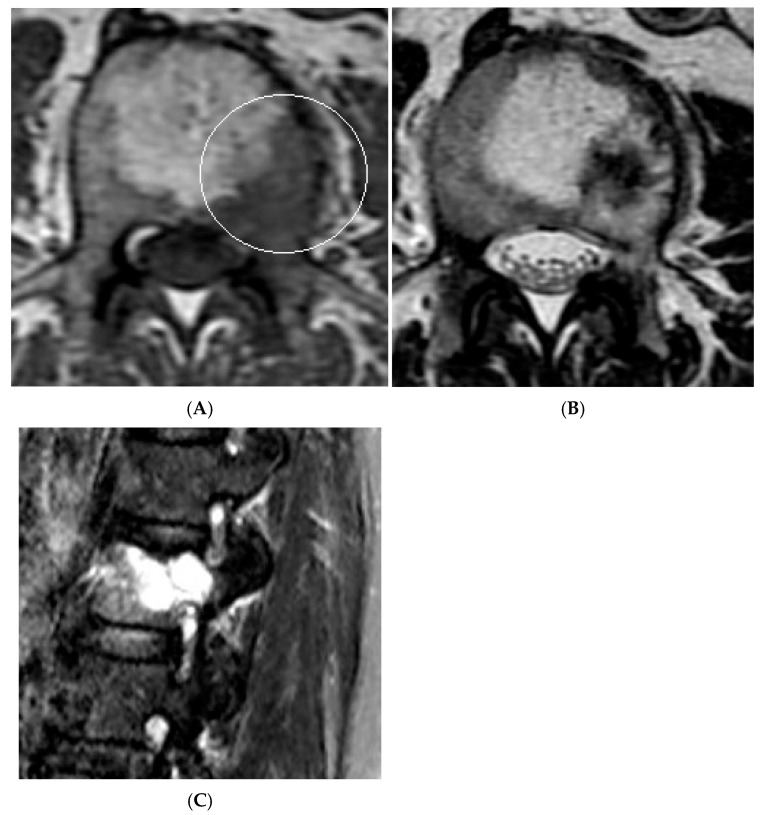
Collision Lesions—Renal cell carcinoma metastases within a haemangioma of the vertebral body, Case 2. (A) Axial T1W MRI with a typical vertebral body haemangioma with an abnormal low T1 signal in its posterolateral aspect with periosteal change (circled). (B) Axial T2W MRI demonstrates the aggressive lesion within and adjacent to the typical haemangioma in image (A). (C) Sagittal STIR MRI—Fat-suppressed haemangioma with a hyperintense (biopsy-proven) metastases in its posterior aspect involving the pedicle.
